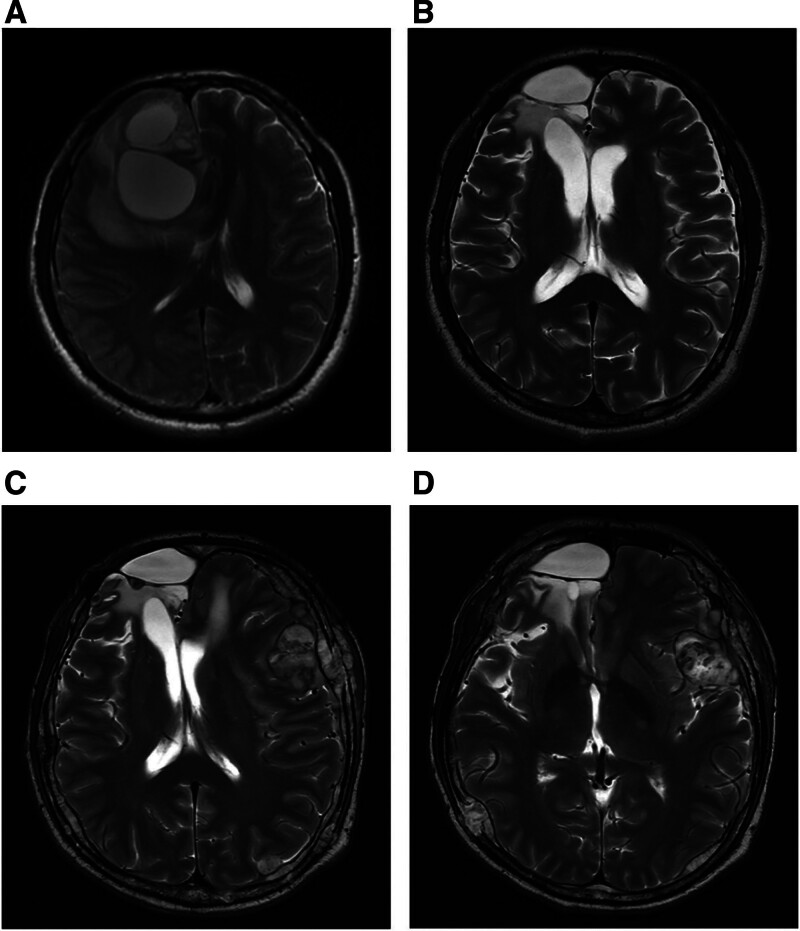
The transverse position T2 images (MRI) of the intracranial primary tumors: (A) Preoperative intracranial lesions on September 2, 2018; (B) No intracranial recurrence was observed on December 13, 2021; (C and D) Metastases were found in meninges, skull, and brain tissues on November 2, 2022. MRI = magnetic resonance imaging.
